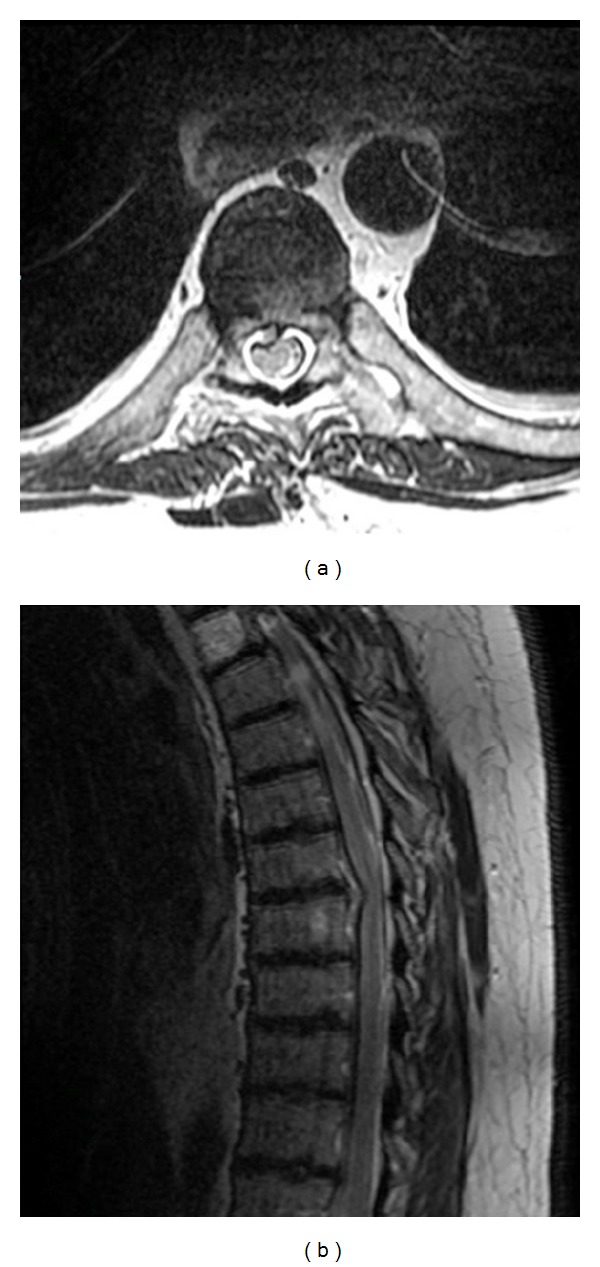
A thoracic spine T2-weighted axial (a) and sagittal (b). MRI reveals a right paramedian disc herniation at the T7-8 level with compression of the spinal cord. The disc appears to be soft and noncalcified.
